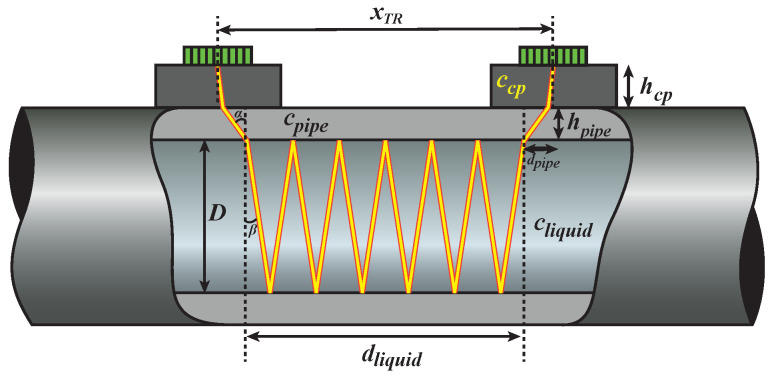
Travel path of an acoustic beam during clamp-on ultrasonic flow measurements. The axial travel path length of the beam is equal to the center-to-center array distance $x_{TR}$. For this particular travel path, n=6.
